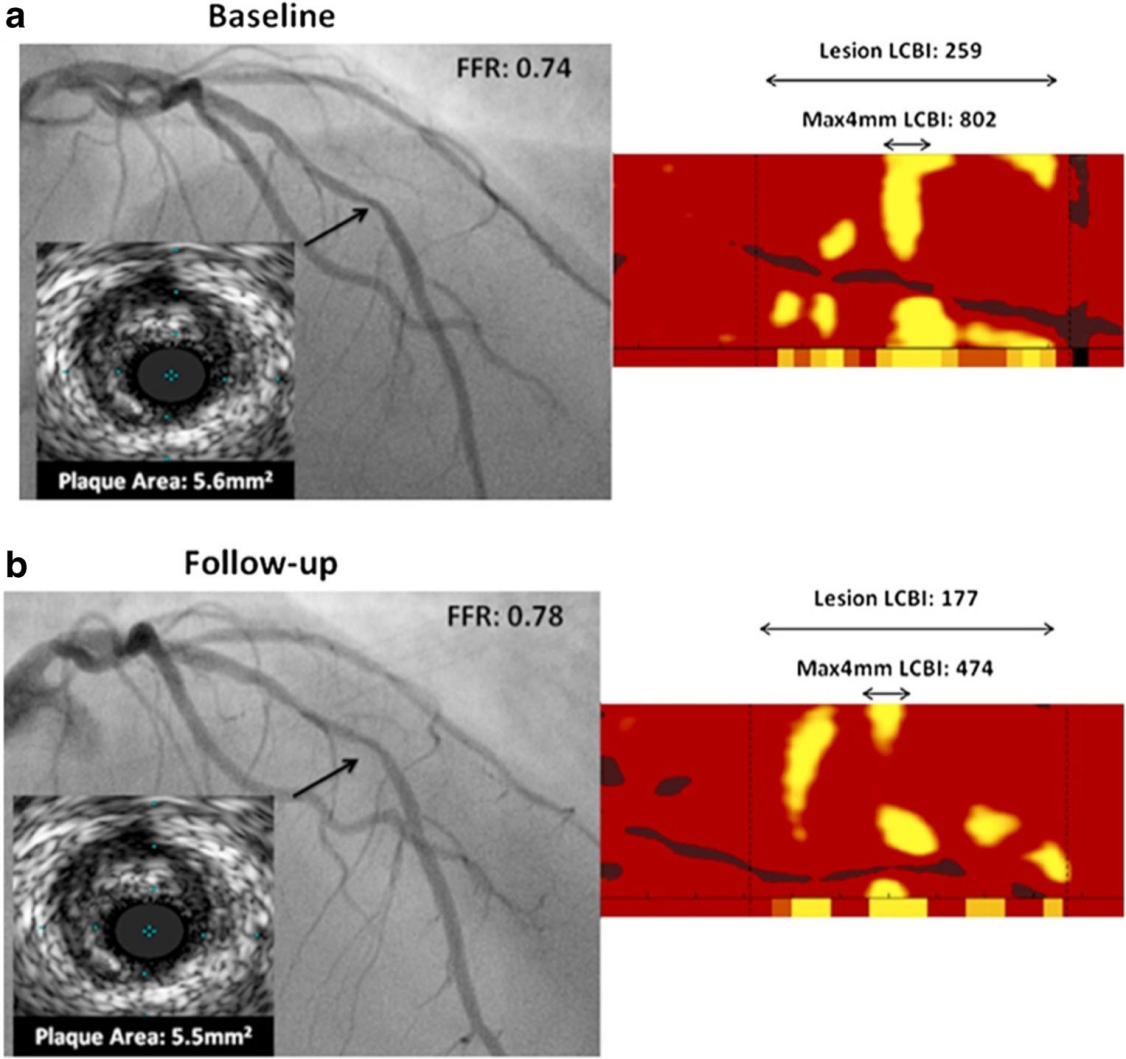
Top panel: On the left, the baseline angiographic image with FRR evaluation and the intravascular ultrasound cross-sectional images of the plaque are shown. On the right, the measurement of lesion lipid core burden index (LCBI) and LCBI at the 4-mm maximal segment (LCBI_4mm_ max) are shown. Bottom panel: On the left, the follow-up angiographic image with FRR re-evaluation and follow-up intravascular ultrasound cross-sectional image are shown. On the right, the reduction of lesion LCBI and LCBI_4mm_ max are shown. (Adapted from: Kini AS et al. J Am Coll Cardiol. 2013 Jul;62(1):21–9, with permission from Elsevier) [27]
